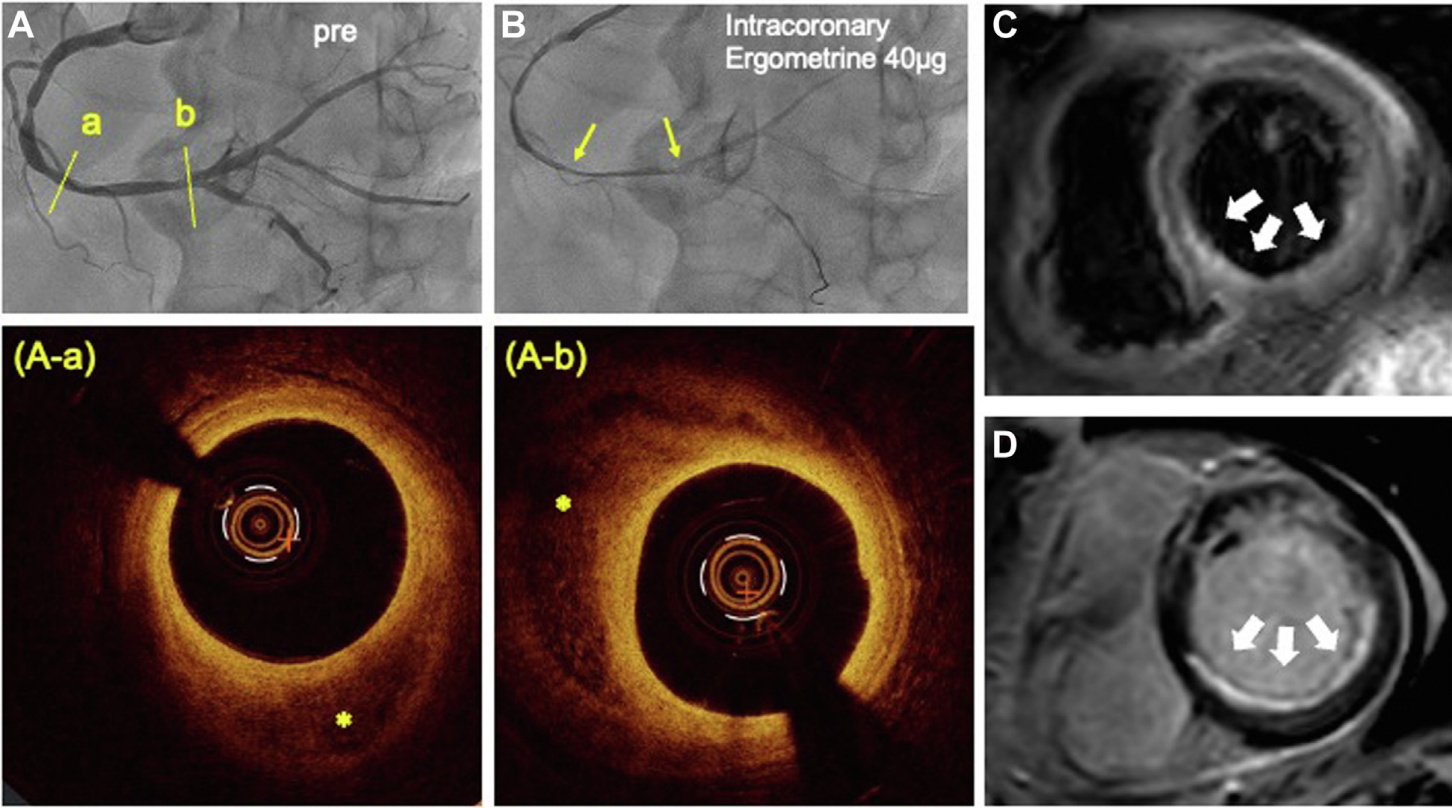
Imaging of Intraplaque Hemorrhage (A) Coronary angiogram of right coronary artery before (pre) an ergonovine provocation test. (B) Severe diffuse stenosis induced by ergometrine (40 μg) was observed in the right coronary artery (a and b, yellow arrows) (A-a and A-b) Optical coherence tomography showed a region of low signal intensity without attenuation, highly suggestive of intraplaque hemorrhage (asterisks). (C and D) Cardiac magnetic resonance showed high signal intensity (white arrows) on T2-weighted images, and subendocardial late gadolinium enhancement was observed in the territory of the right coronary artery.
